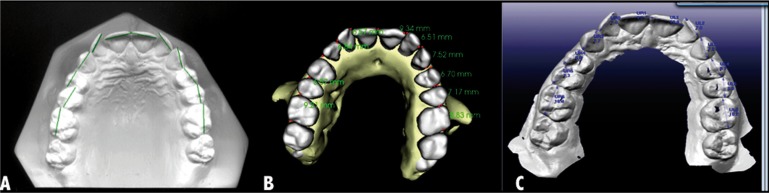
Measurement of mesiodistal tooth sizes: 2D digital (A), CBCT (B) and ILS models (C).
